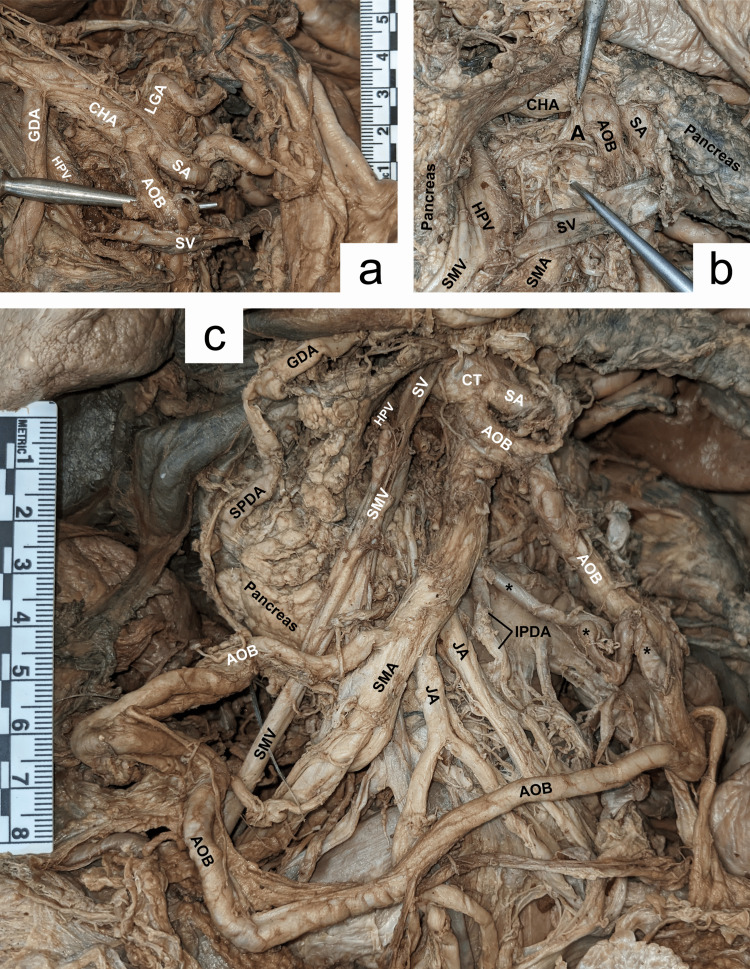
A novel variant of the AOB (a) The AOB, left gastric artery, splenic artery, and common hepatic artery emerging from the celiac trunk, which is located at the center of the four branches. (b) The celiac trunk has been reflected superiorly using a probe to reveal the abdominal aorta, partially obscured by the autonomic plexus; the lower probe marks the approximate origin of the SMA. The inferior vena cava is not visible; for reference, it is located posterior to the common hepatic artery and hepatic portal vein. (c) The full length of the AOB, from the celiac trunk to the SMA. The pancreas and splenic vein have been reflected superiorly; the transverse colon has been pulled inferiorly and is only visible at the lower right and left corners of the image. The branch of the AOB that connects with the IPDA is marked with asterisks. A, aorta; AOB, arc of Bühler; CHA, common hepatic artery; CT, celiac trunk; GDA, gastroduodenal artery; HPV, hepatic portal vein; IPDA, inferior pancreaticoduodenal artery; JA, jejunal arteries; LGA, left gastric artery; SA, splenic artery; SMA, superior mesenteric artery; SMV, superior mesenteric vein; SPDA, superior pancreaticoduodenal artery; SV, splenic vein.
